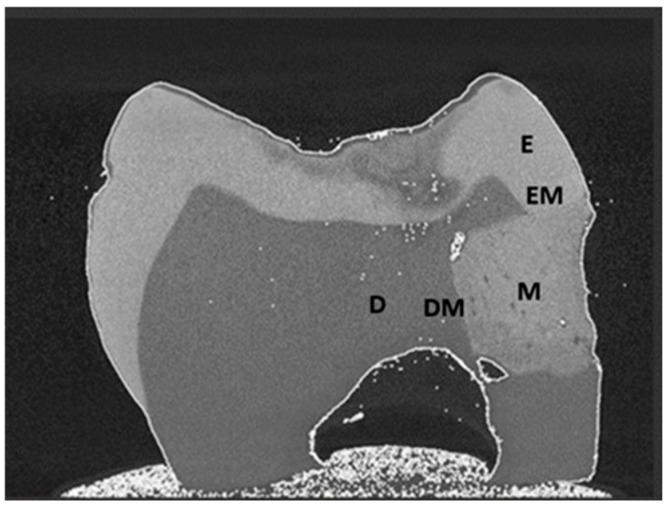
Computed tomography (CT) scan of a sample showing the locations where EDS analyses were conducted: E—enamel distant from the material; D—dentine distant from the material; M—restorative material; EM—enamel adjacent to the material; DM—dentine adjacent to the material.
